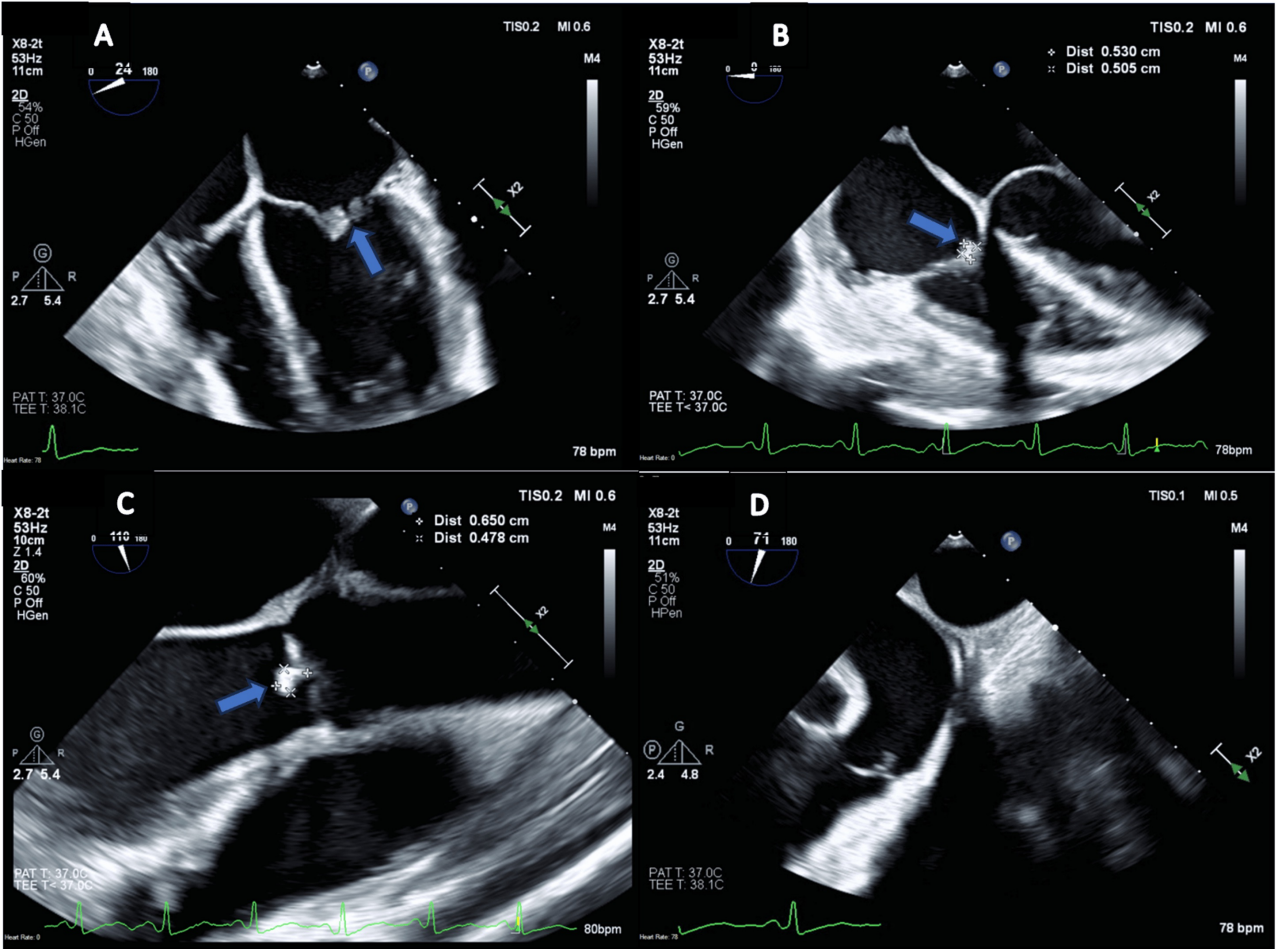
Echocardiographic Findings (A) Mitral valve vegetation; (B) Tricuspid valve vegetation; (C) Aortic valve vegetation; (D) Normal pulmonic valve
